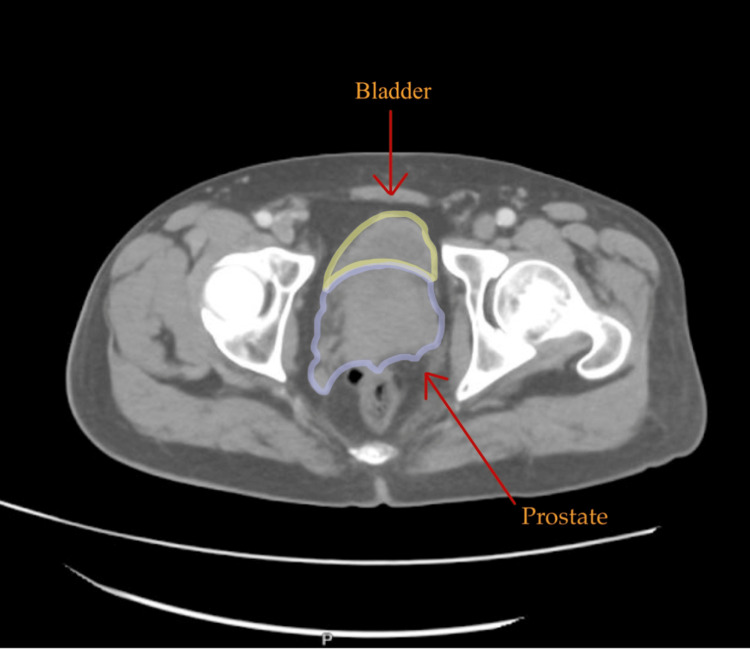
CT abdomen pelvis axial view demonstrates an enlarged prostate The bladder was not well-distended for evaluation.
